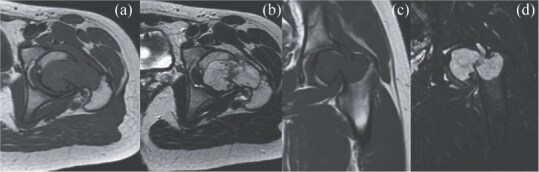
MRI findings. (a) Coronal T1 weighted view, (b) coronal T2 weighted view, (c) sagittal T1 weighted view, and (d) sagittal STIR view.
